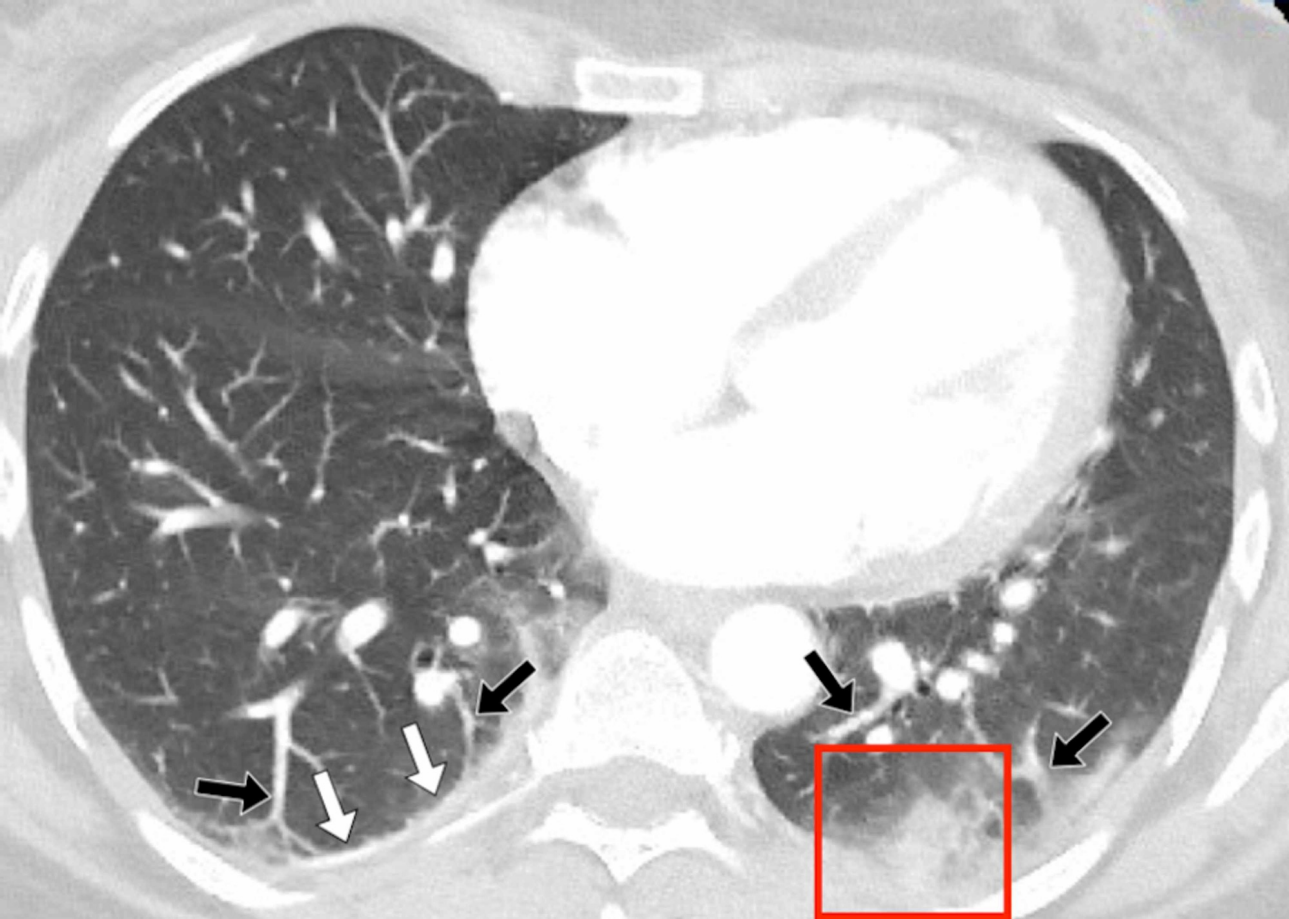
Patient 2 A 44-year-old female patient presented with persistent shortness of breath, pleuretic chest pain, and a raised D-dimer of more than 1400. A pulmonary embolus was suspected, and the patient was investigated with a CT pulmonary angiogram (CTPA). The CTPA showed no embolus; however, the patient did have features of COVID-19, which would account for her symptoms. Figure 3a demonstrates an axial, contrast-enhanced, CT image through the thorax, in lung windows. There was evidence of left basal consolidation (red box), right basal pleural thickening (white arrows), and basal vascular engorgement (black arrows).
